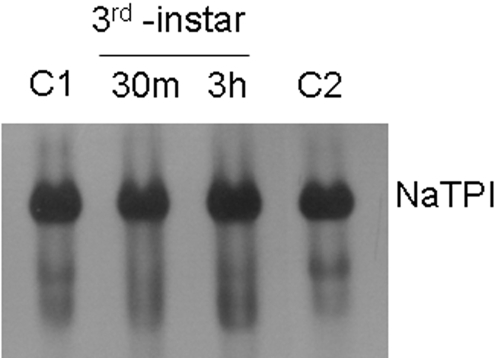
Stability of NaTPI against trypsin of *M. sexta* larvae. Leaf extracts of WT containing NaTPI incubated with 2 trypsin inhibitor units of third-instar gut proteinase for 0.5 h or 3 h at 30°C. Two controls were used: C1 (not incubated) and C2 (incubated without gut proteinases for 3h). Mixtures were resolved on 10% PAGE and then visualized for trypsin inhibitor activity using gel X-ray film contact print technique [60].
